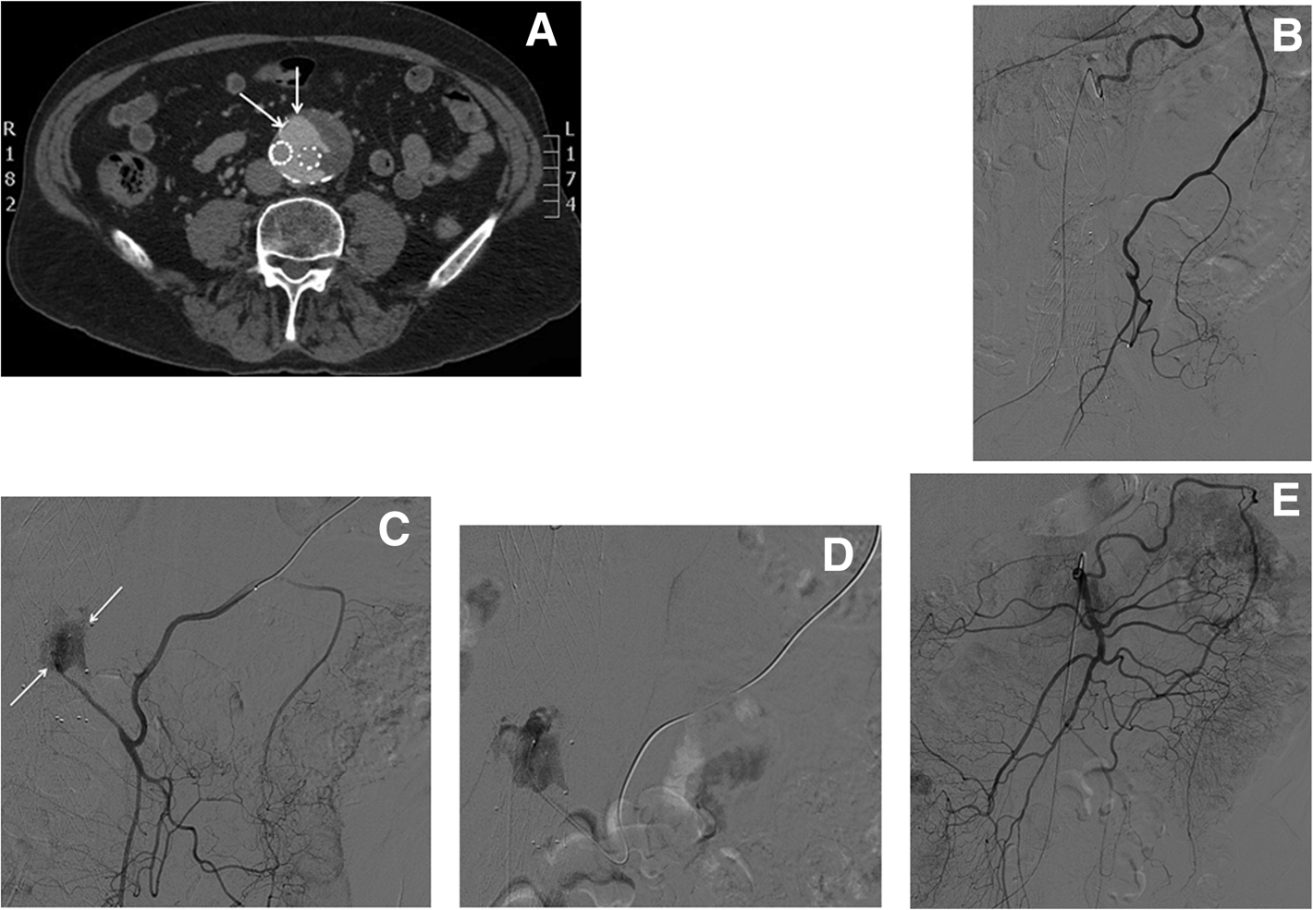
Type II endoleak embolization. a Contrast-enhanced CT in arterial phase shows the presence of a type II endoleak post EVAR (white arrows). b Transfemoral diagnostic DSA of the superior mesenteric artery shows hypertrophy of the Riolano arch. c Further diagnostic DSA performed by a coaxial microcatheter advanced in the Riolano arc confirms the endoleak opacification (white arrows) sustained by the inferior mesenteric artery stump. d Microcatheter advancement into the aneurysmatic sac and Squid embolization. e Final angiographic control shows complete endoleak exclusion
